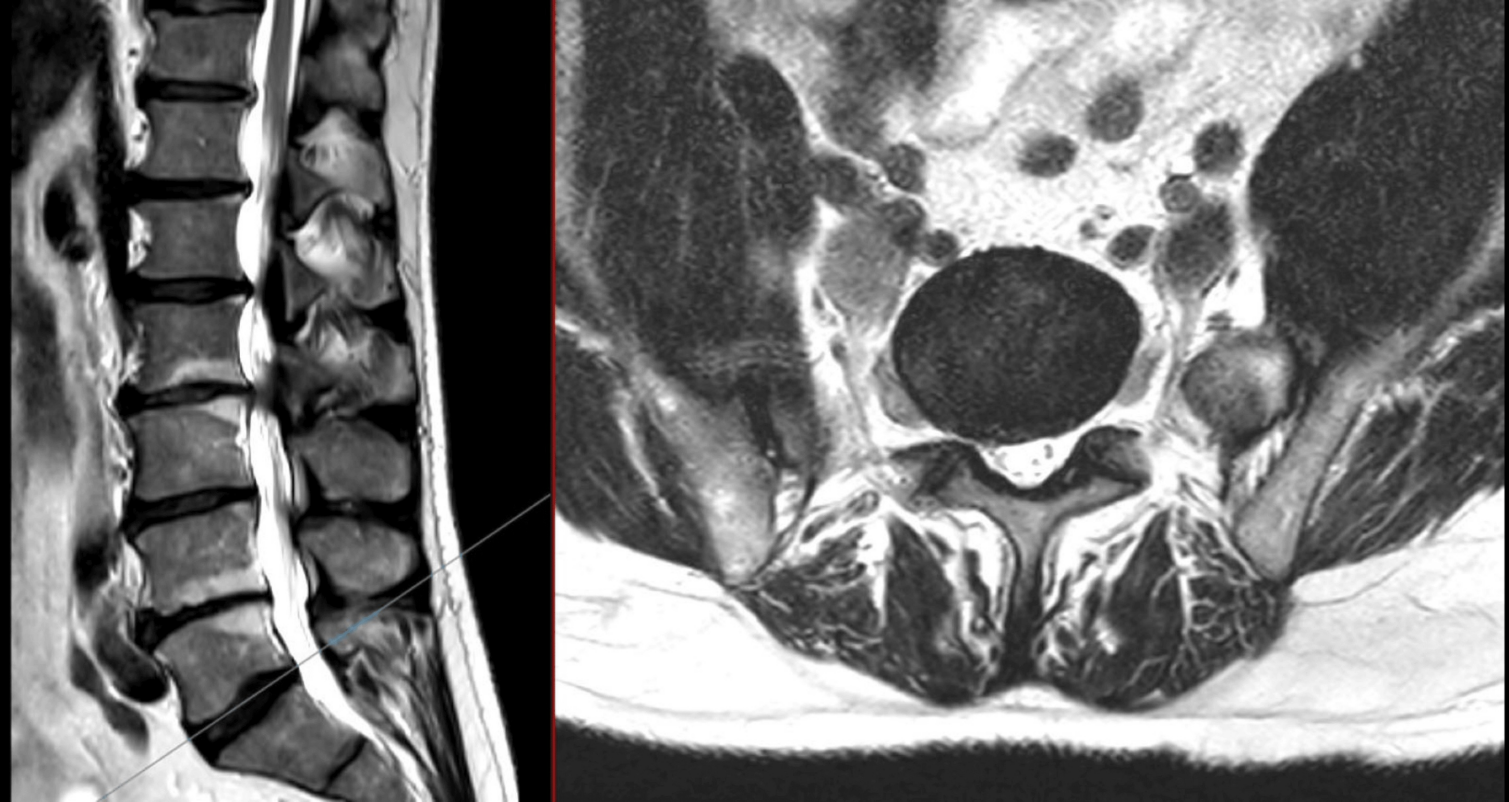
T2 MRI of the lumbar spine: sagittal (left) and axial (right) at L5/S1 Neither compression of the S1 nerve roots nor cauda equina nerve roots at this level was present.
